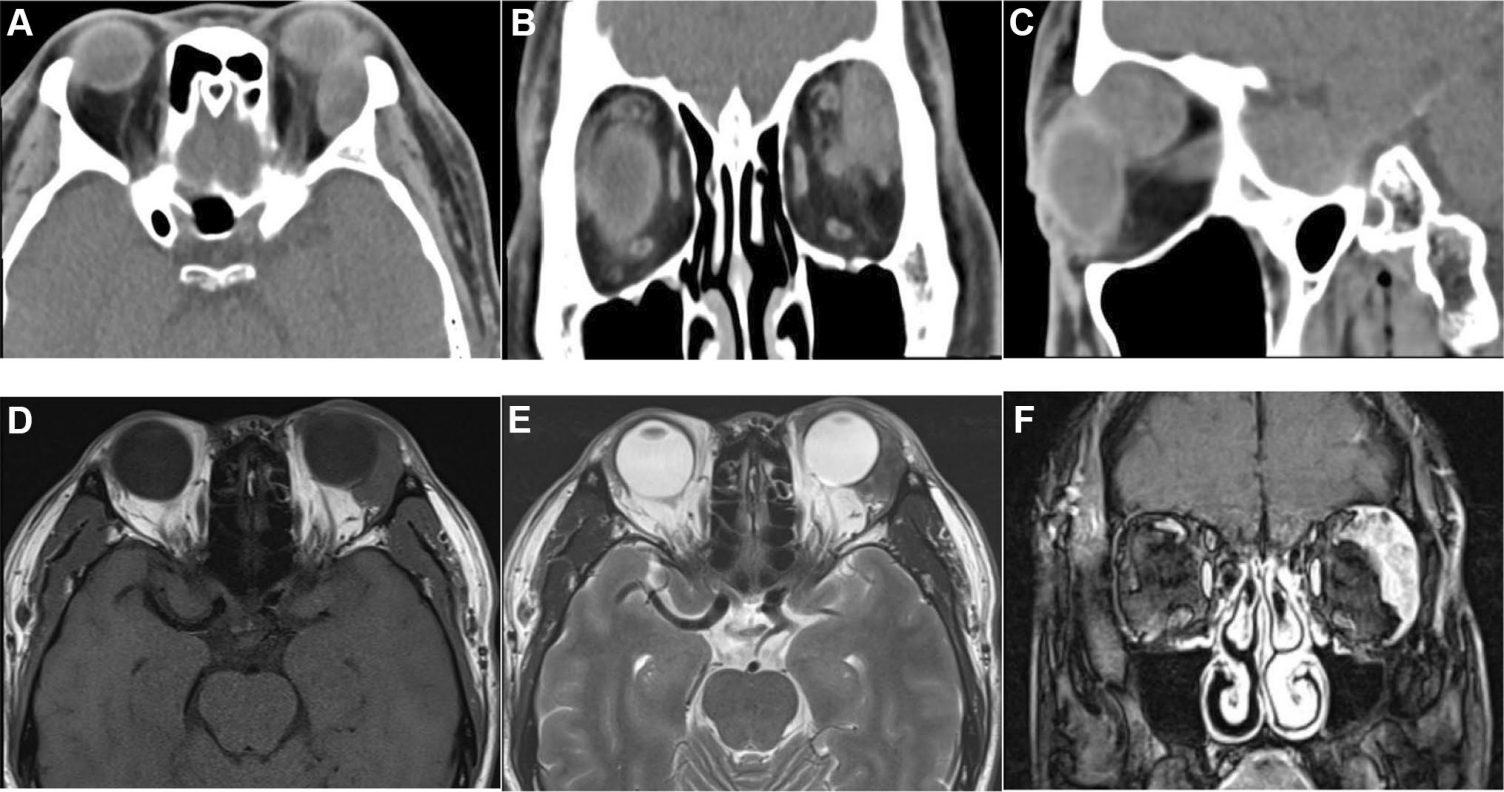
Imaging findings of lacrimal duct adenocarcinoma. A The lacrimal gland mass of the left eye had clear boundaries and uneven density. B The left lacrimal gland mass compressed the eyeball. C The left lacrimal gland mass compressed the extraocular muscle and bone wall. D A mass in the left lacrimal gland with blurred boundaries and indistinct boundaries from surrounding tissue, and a medium T1-weighted images. E A slightly hypointense signal on T2-weighted images. F The tumor showed obvious enhancement on enhanced scan
